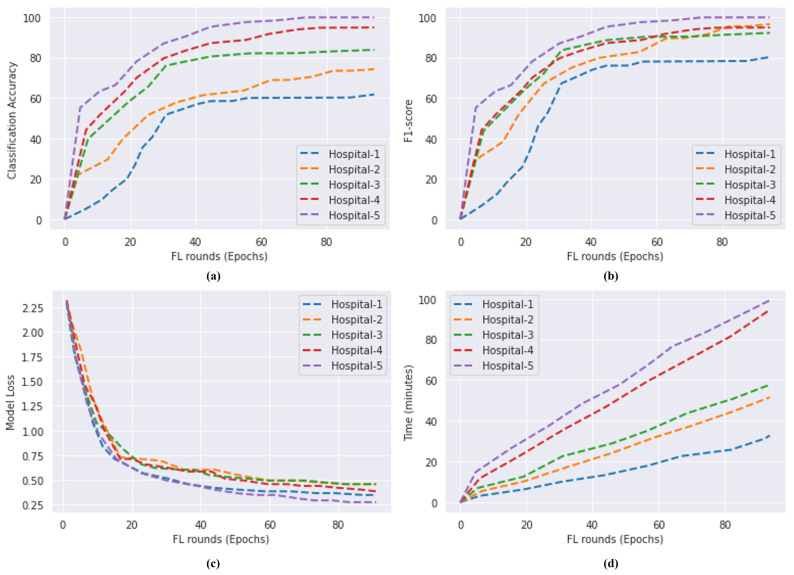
A result achieved by the proposed blockchain-based FL model. (a) Accuracy of the proposed model on COVID-19 CT scan dataset for five different hospitals. (b) F1-score of the proposed model; (c) model loss on COVID-19 CT scan dataset five different hospitals. (d) Time of COVID-19 CT scan dataset for five different hospitals using FL.
